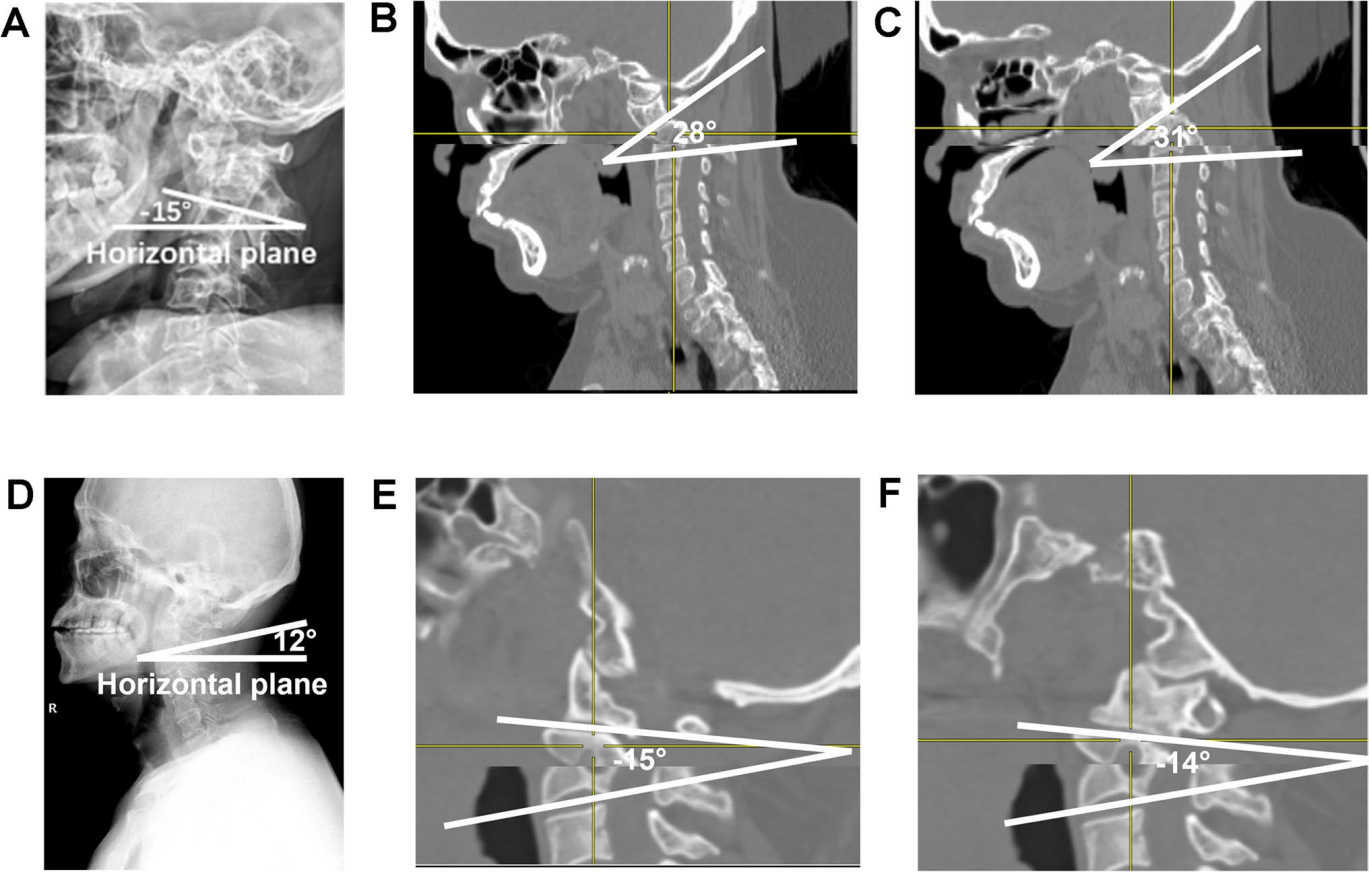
Measurement of ASFEA in AAD patients. Herein, C2S + ASFEA = ASFS. A Cervical X-ray of an AD patient in a neutral position and measurement of C2S. B Measurement of left ASFEA in an AD patient. C Measurement of right ASFEA in an AD patient; (left ASFEA (28°) + right ASFEA (31°))/2 + C2S (− 15°)) = ASFS (14.5°). D of Cervical X-ray of a PD patient in a neutral position and measurement of C2S. E Measurement of left ASFEA in a PD patient. F Measurement of right ASFEA in a PD patient; (left ASFEA (− 15°) + right ASFEA (− 14°))/2 + C2S (12°)) = ASFS (− 2.5°)
